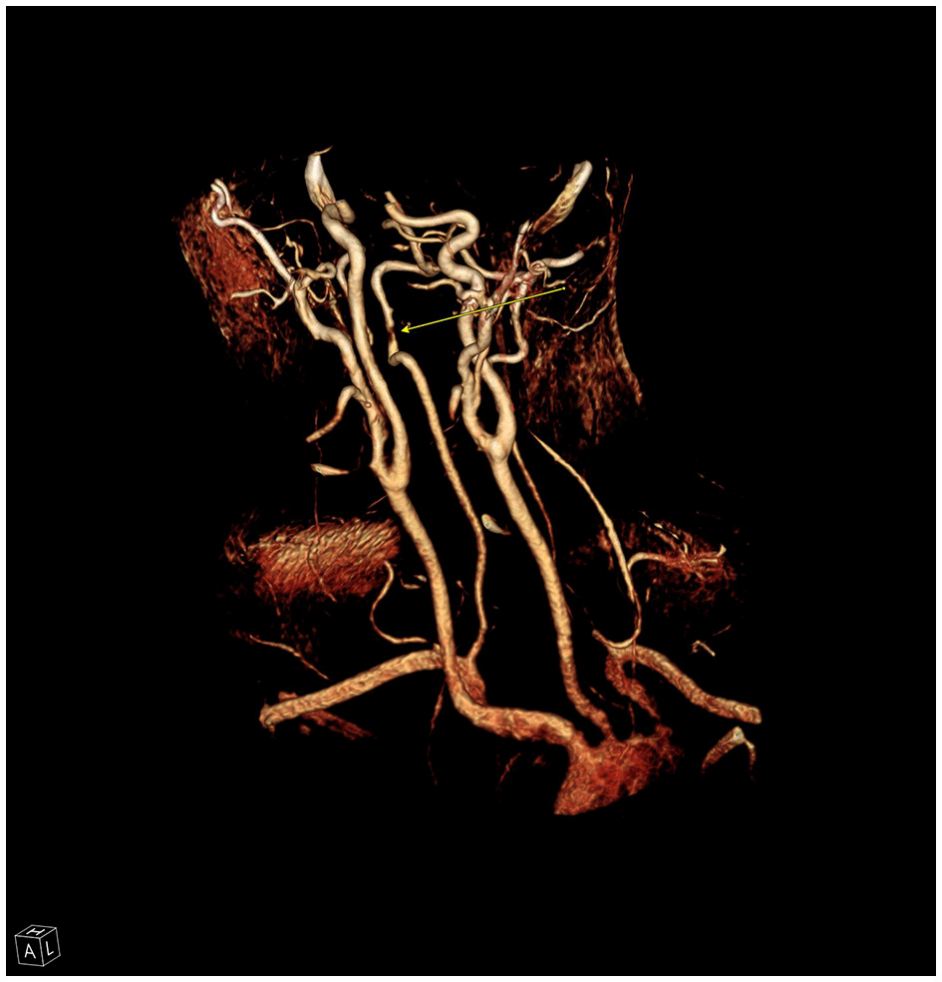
Reconstruction from CT angiography of the neck demonstrating high grade stenosis (arrow) of the right vertebral artery due to dissection and intraluminal thrombus.
